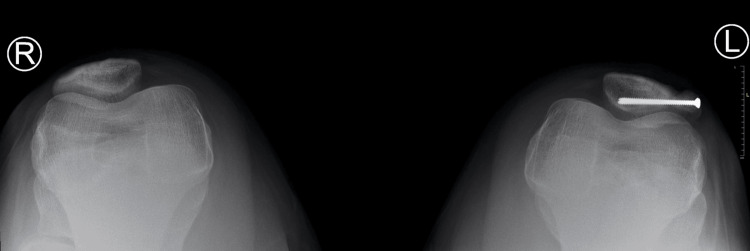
12-month postoperative X-ray B/L knee sunrise view B/L: Bilateral.
